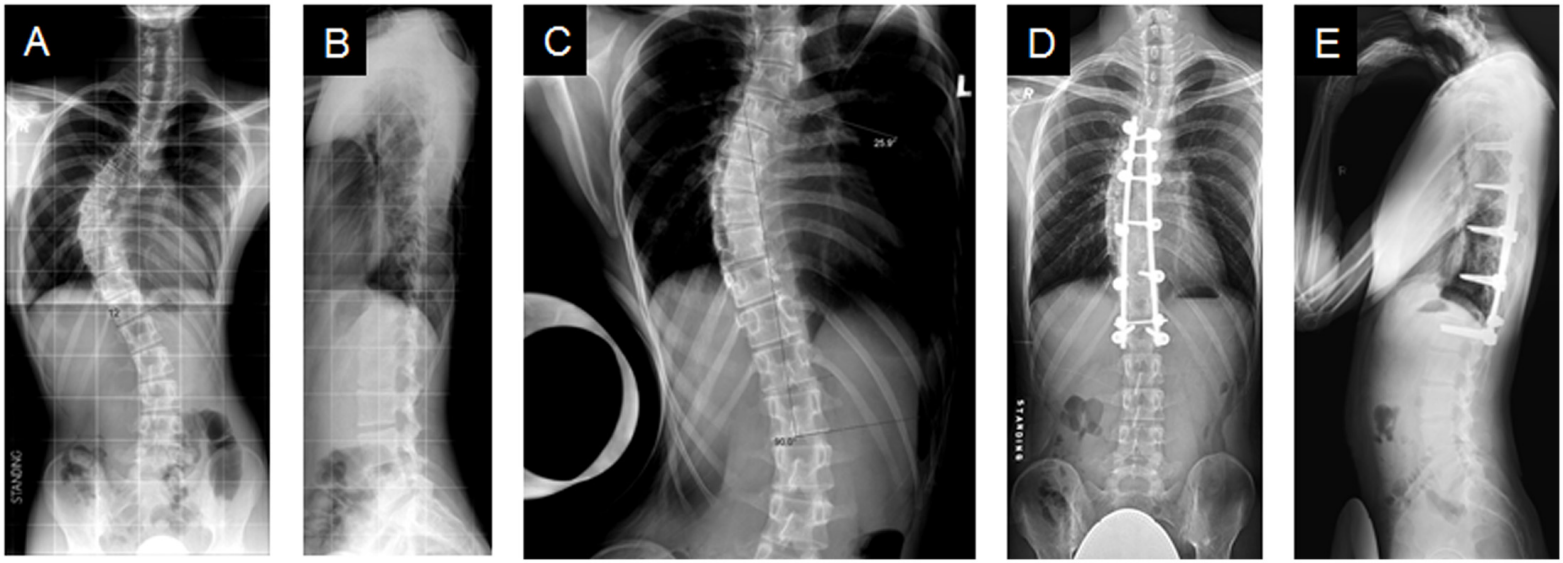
Saved distal fusion levels. A case where one level was saved. (A) A male AIS patient with a preoperative standing coronal Cobb angle of 61.6 degrees from T5-T12. (B) His standing sagittal Cobb angle from T5-T12 was 5.1 degrees. (C) Fulcrum bending radiograph demonstrated a curve of 31.3 degrees. Last follow-up (D) standing coronal Cobb angle was 26.8 degrees and (E) standing sagittal Cobb angle was 4.5 degrees.
